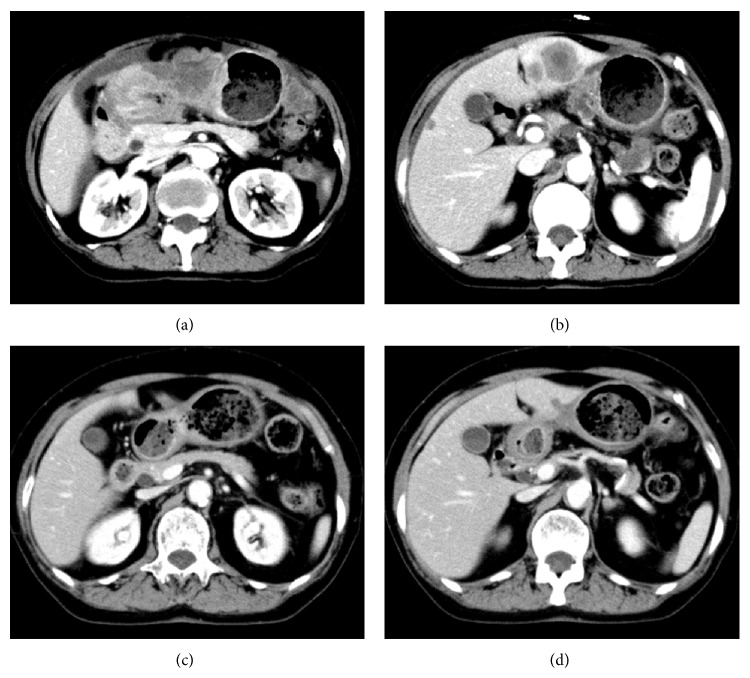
Contrast-enhanced computed tomography (CT) findings. (a) and (b) show the CT at presentation. (a) The wall of the lower part of the stomach is markedly thickened, and the density of the surrounding fat tissue is increased, forming an 80 × 45 mm mass. (b) Two nodules are detected in the lateral segment of the liver, and these are directly contiguous from the gastric mass. (c) and (d) show the CT findings after completion of the 11th course of chemotherapy (capecitabine + CDDP + trastuzumab). (c) The gastric mass is markedly decreased. (d) Only a small nodule in the lateral segment of the liver is detected.
